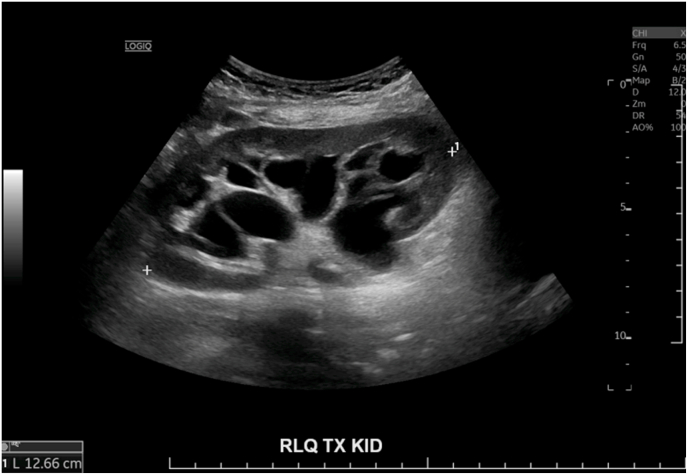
Ultrasound of allograft kidney mimicking hydronephrosis. (July 2024).
